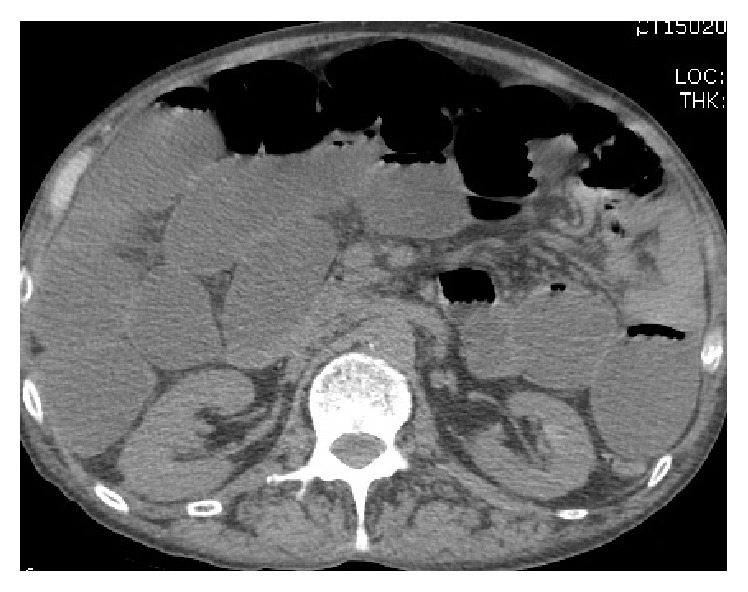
CT scan of the abdomen showing small bowel dilatation.
